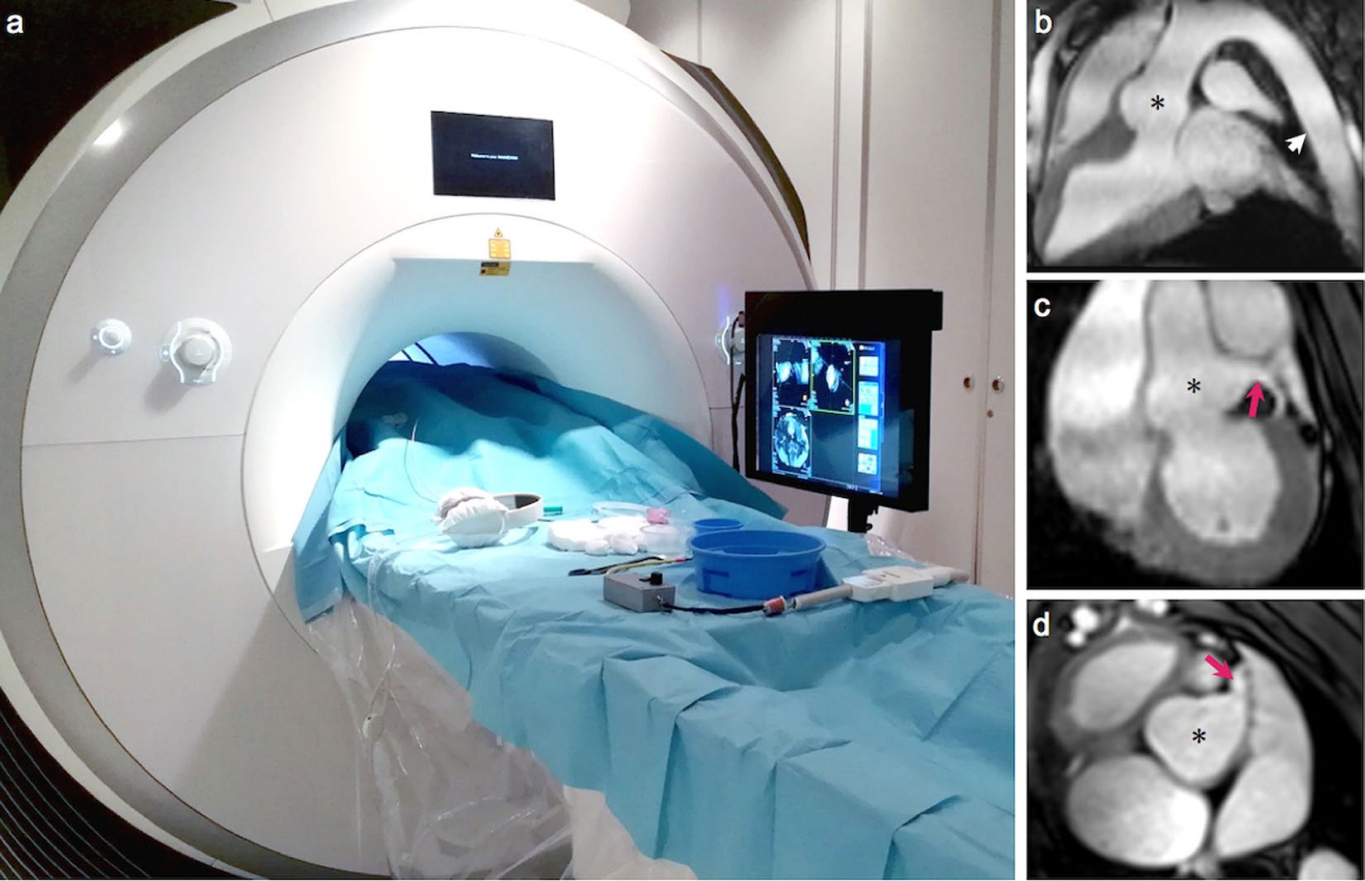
Interventional setup. (a) Setup of an interventional MR-suite for real-time intervention using a 3 Tesla MRI system (Siemens AG, Erlangen, Germany) and a commercially available non-magnetic and RF-shielded in-room monitor. Pre-defined road maps, reconstructed from a 3D whole heart data set, were used to increase the speed for navigation and anticipate laborious image adjustments. Image planes were (b) an “aortic arch view” depicting the arch from the descending aorta (white arrow) to the aortic root (*) (c) the “aortic root view” showing the aortic root (*) in an oblique coronal view including the left coronary ostium (red arrow) and (d) the “aortic root short axis view” oriented perpendicular to the image in (c).
